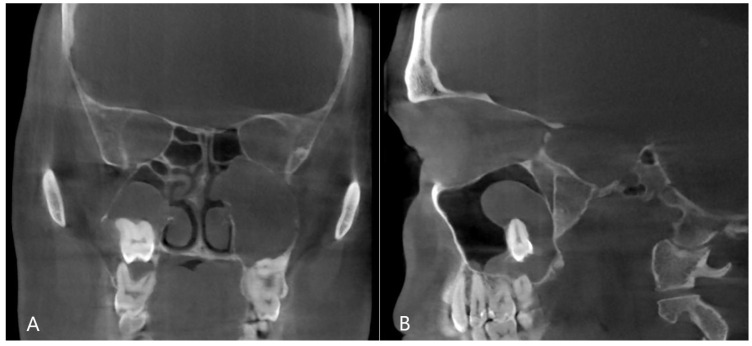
CBCT image of odontogenic cyst with calcified capsule, highly impacted upper wisdom tooth in the right maxillary sinus. Left chronic odontogenic sinusitis caused by impacted left upper wisdom. ((A): coronal, (B): sagittal view).
